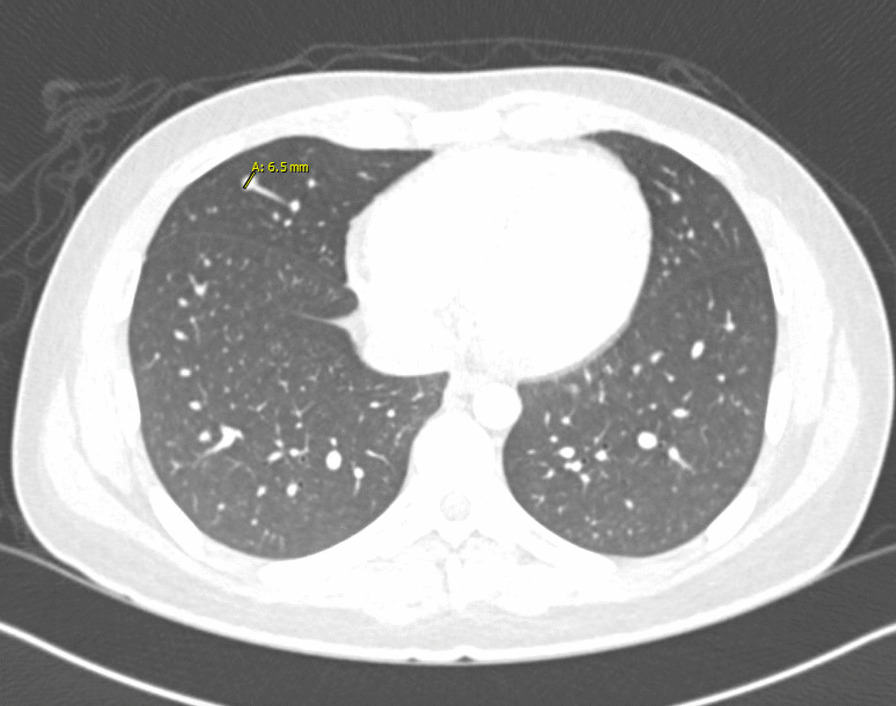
Chest CT imaging showing a 6.5mm right middle lobe nodulemaculopapular lesions
